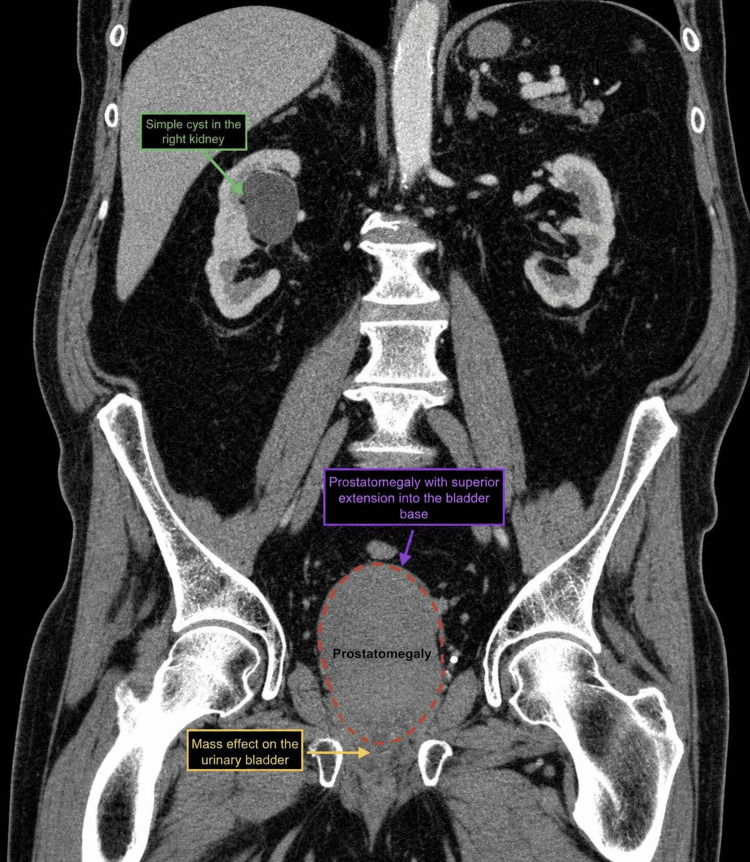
Coronal contrast-enhanced CT Image showing massive enlargement of the prostate gland with mass effect on the urinary bladder. Simple cyst in the right kidney.
